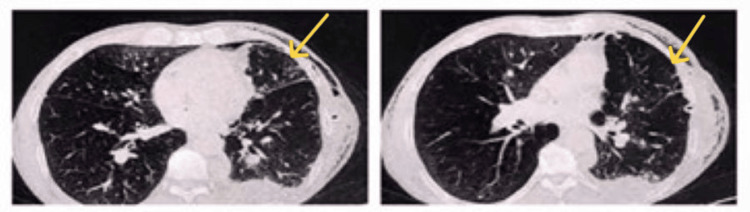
A follow-up thoracic computed tomography Follow-up chest computed tomography obtained on postoperative day 5 shows the appropriate re-expansion of the lung, with the left lung adequately opposed to the thoracic wall (yellow arrows)
